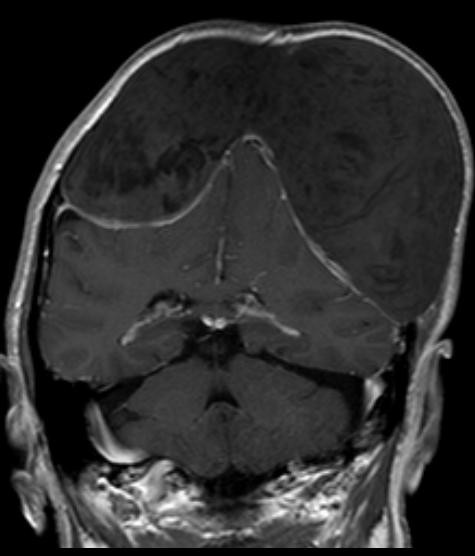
Magnetic resonance imaging T1 weighted image past gadolinium shows a thickening of the dura, but no enhancement within the epidermoid tumor.
